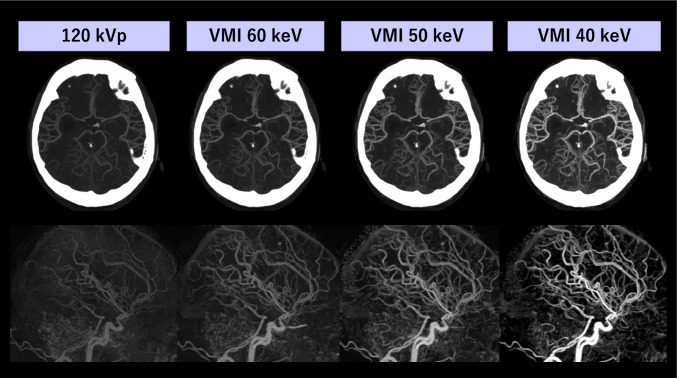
A 40-year-old man with subarachnoid hemorrhage underwent cranial CTA for treatment planning using dual-layer detector scanner. An ICM dose of 25 mL (370 mgI/mL, diluted with 25 mL of normal saline) was injected over 10 s. Conventional 120-kVp imaging resulted in poor arterial enhancement. Arterial attenuation gradually increased as VMI energy decreased, with excellent visualization of intracranial arteries observed at 40 keV VMI. The short ICM injection duration also helped to minimize venous contamination at the cavernous sinus
